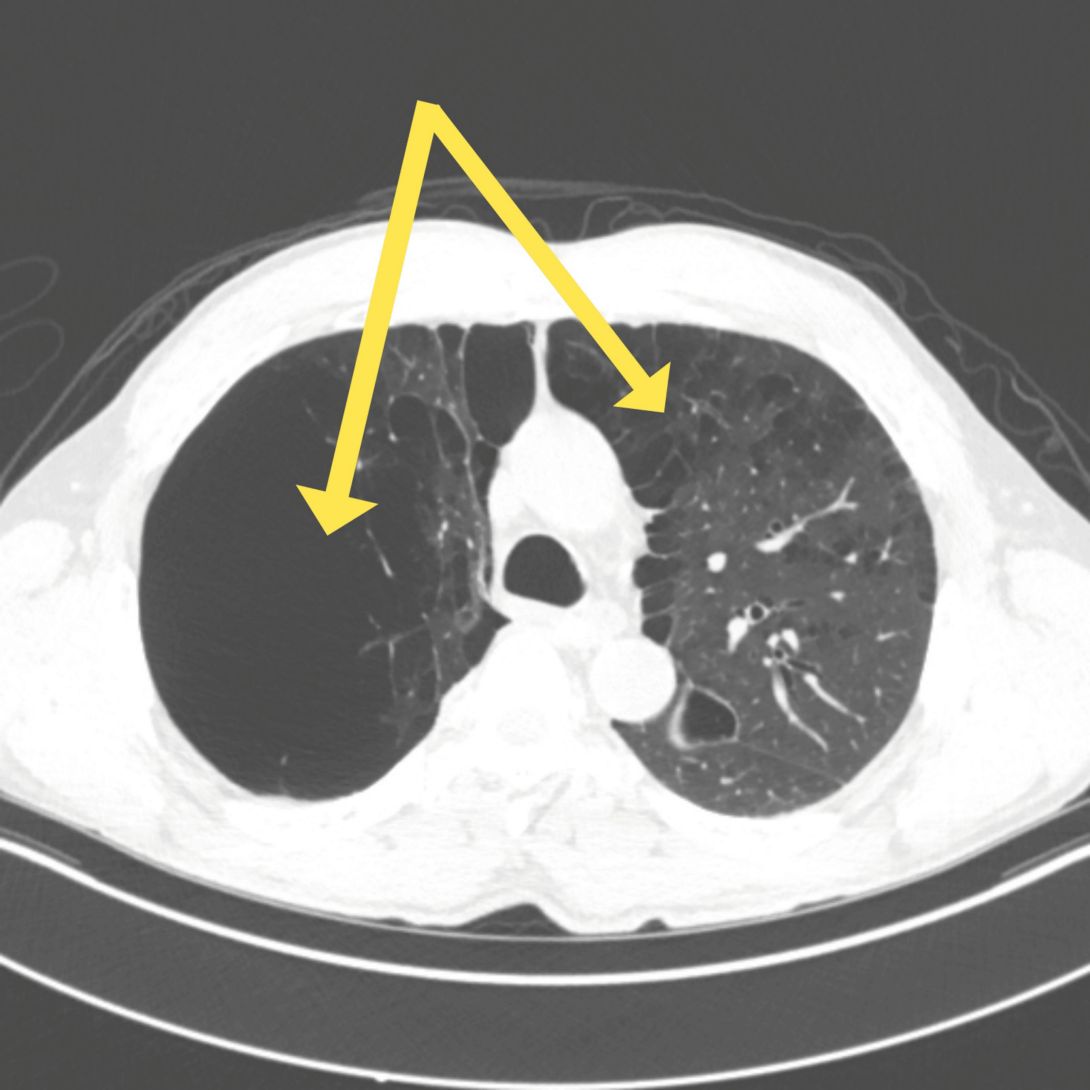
Preoperative axial chest computed tomography (CT) scan showing extensive bullous emphysema. The yellow arrows indicate giant bilateral bullae occupying the majority of the right upper and middle lobes.
